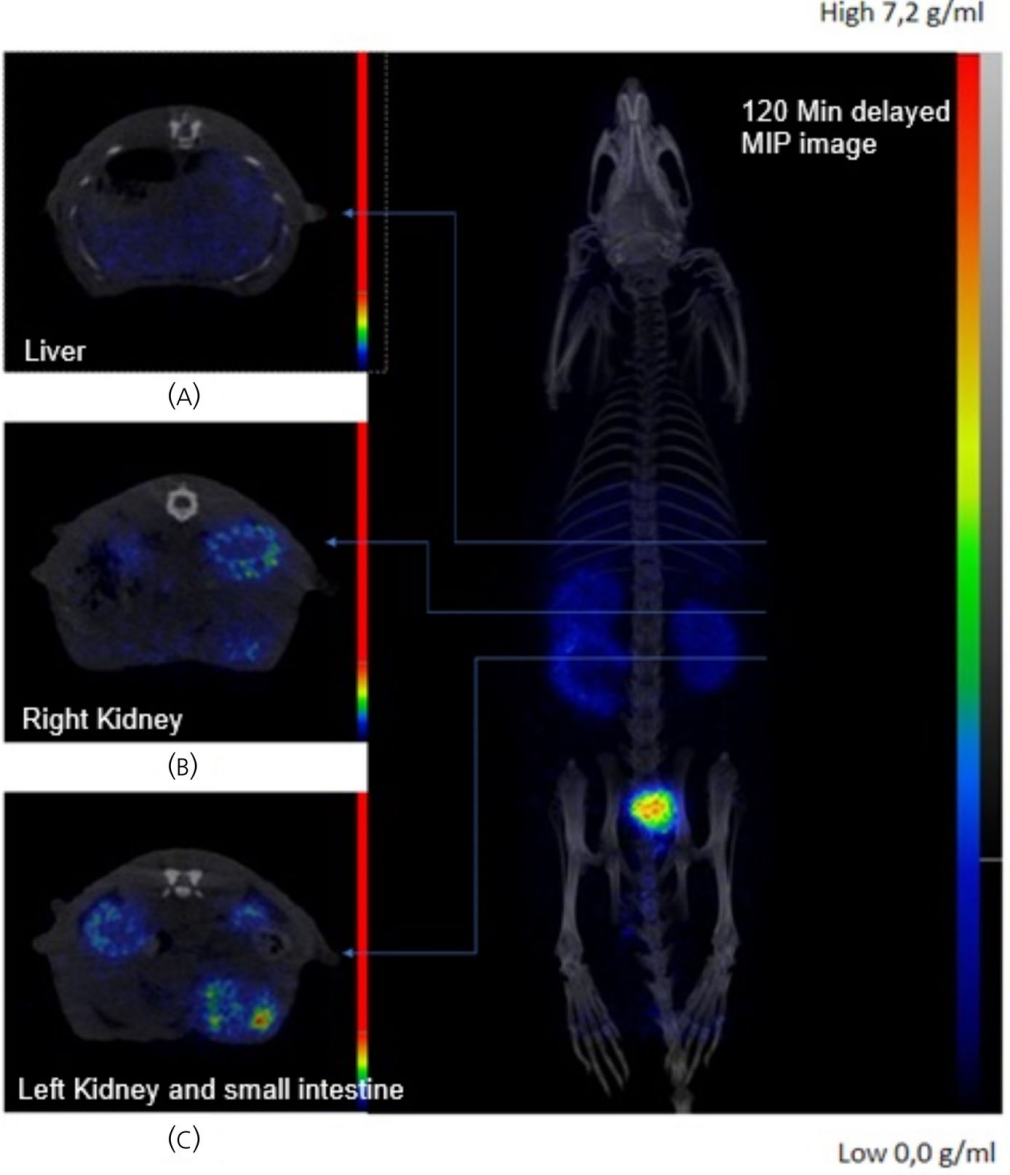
An example of a full body image (prone) in maximum intensity projection (right panel) from a 120‐min delayed micro‐PET/CT acquisition (right) with cross‐sectional micro‐PET/CT image slices (left panel) demonstrating noticeable radioactivity in (A) liver, (B) right kidney, (C) left kidney and small intestine. CT, computerised tomography; PET, positron emission tomography.
